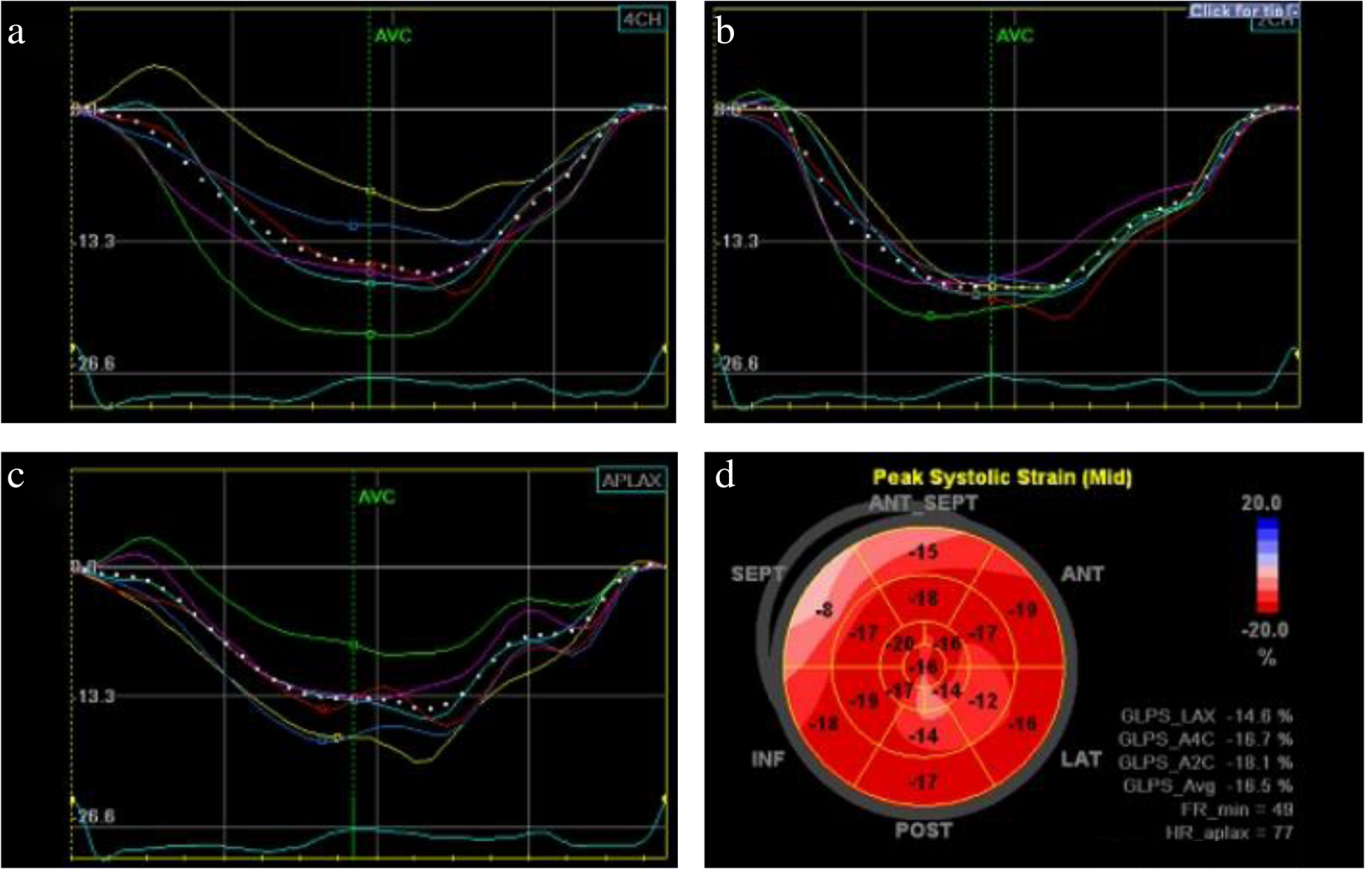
Longitudinal strain curves from four-chamber (a), two-chamber (b), and apical long-axis (c) views obtained by two-dimensional transthoracic echocardiography approximately 2 months after admission are used to generate bull’s-eye summary of the entire left ventricle (d). The bull’s-eye plot shows near normal left ventricular wall motion
